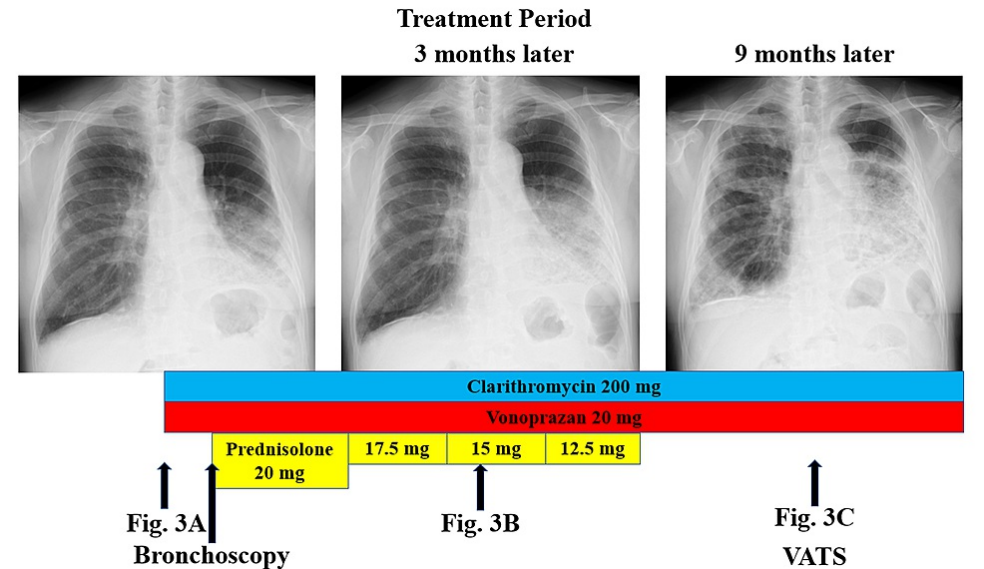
Clinical course of the patient. Although clarithromycin 200 mg and vonoprazan 20 mg were started for the possibility of chronic bronchitis and bronchiectasis due to aspiration or some other cause, no improvement was observed. BALF from left B5 showed elevated lymphocytes, and treatment with prednisolone 20 mg was started for the possibility of organizing pneumonia. The chest X-ray showed multiple GGOs that spread over time, and shortness of breath on exertion progressed.
